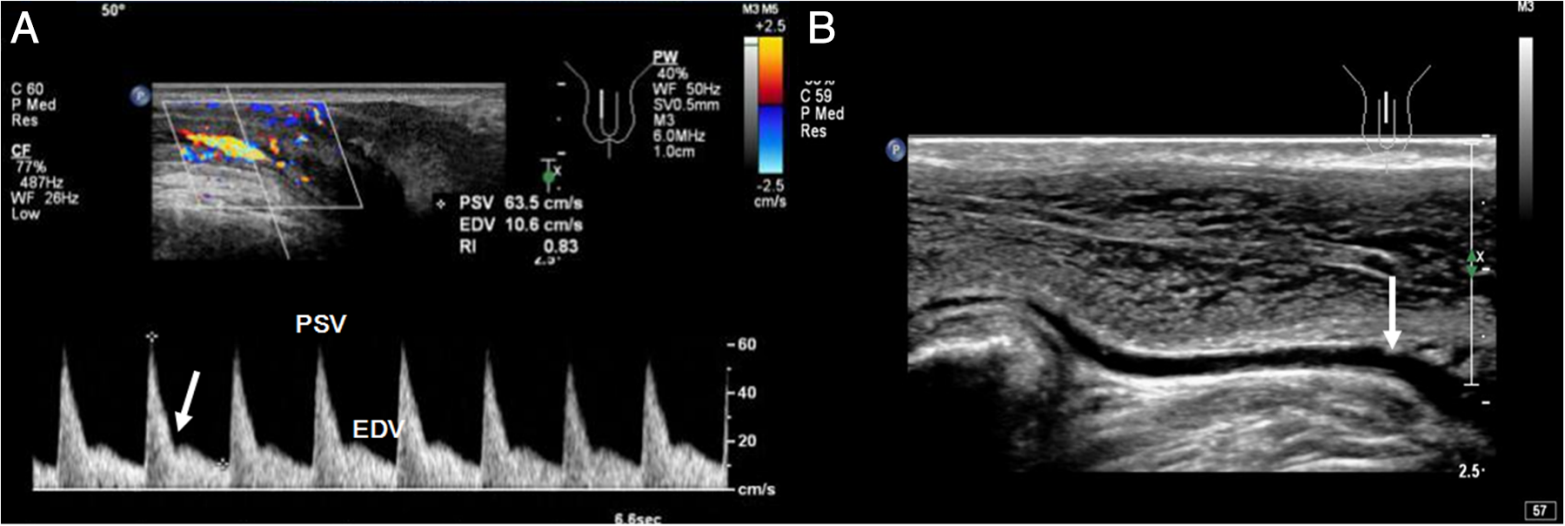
Normal ultrasound images in a 24‐year‐old man. A, Blood flow spectrum of the cavernous artery at the end of phase II with a characteristic of a waveform notch (arrow) at end systole. B, Measurement site (arrow) of the deep dorsal vein at the base of the penis.
